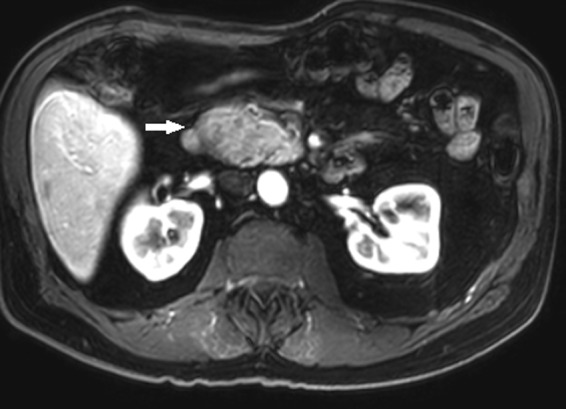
T1-weighted arterial phase contrast-enhanced axial MRI demonstrates heterogeneous enhancement of the well-defined mass (arrow).
